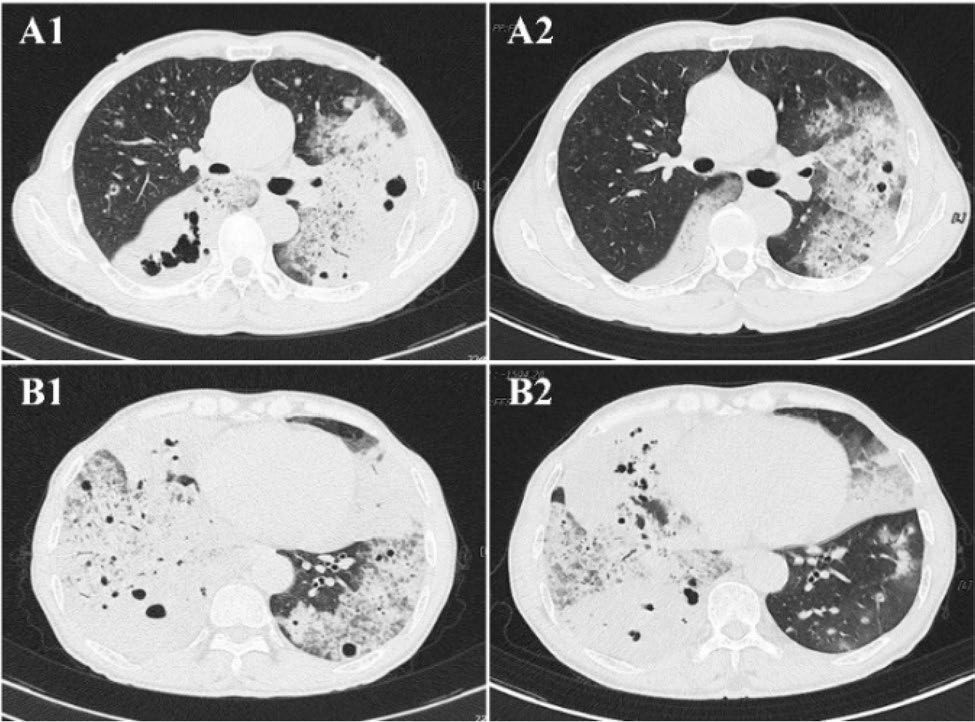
Serial chest CT comparison showing disease progression between first (A2, B2) and second admission (A1, B1), with increased extent of bilateral lung lesions, greater number of cavities, and enlargement of nodules
